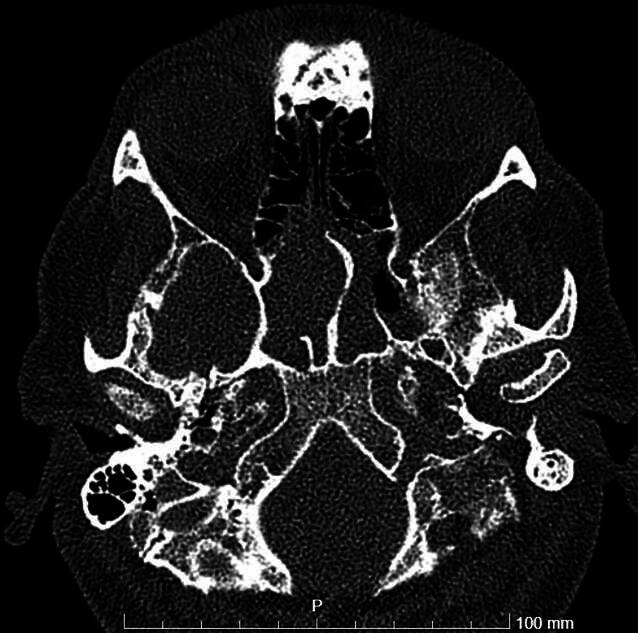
CT der Nasennebenhöhlen, transversale Schicht: *rechts* komplett, *links* teilverschatteter Sinus sphenoidalis
